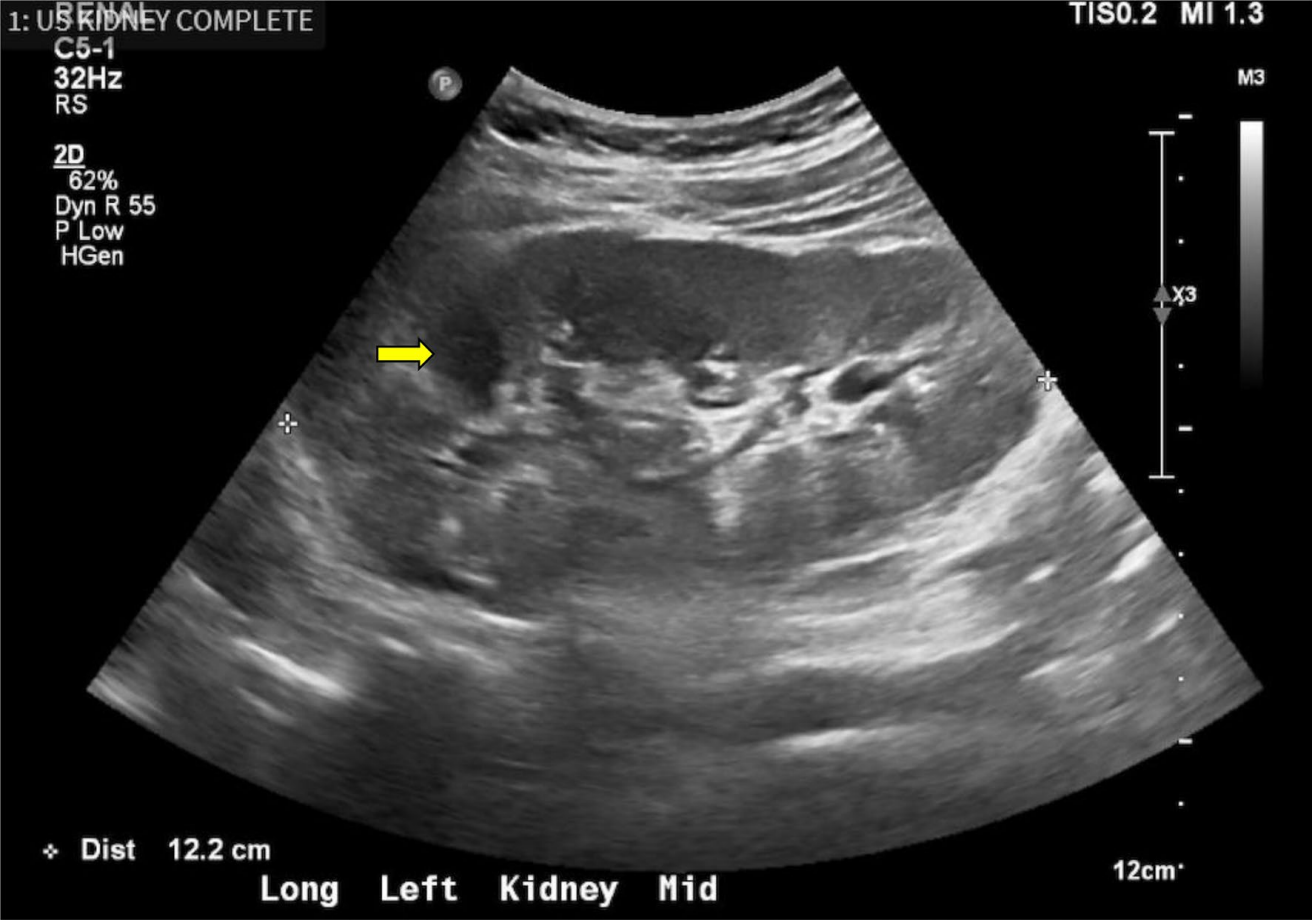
Longitudinal ultrasound image of the left kidney (coronal image)
